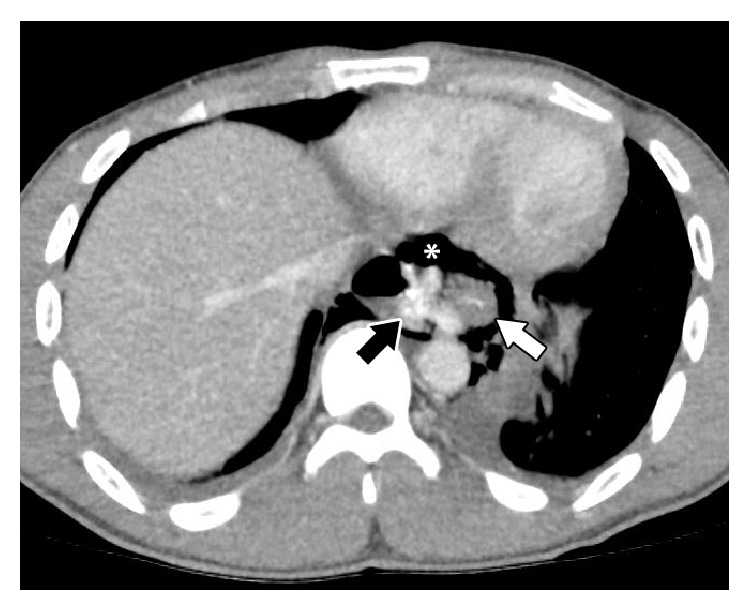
Chest computed tomography (CT) scan revealing a distal esophageal rupture with an oral contrast leak (indicated by the black arrow) from the ruptured esophagus (indicated by the white arrow), in addition to pneumomediastinum (asterisk) and left-sided pleural effusion.
